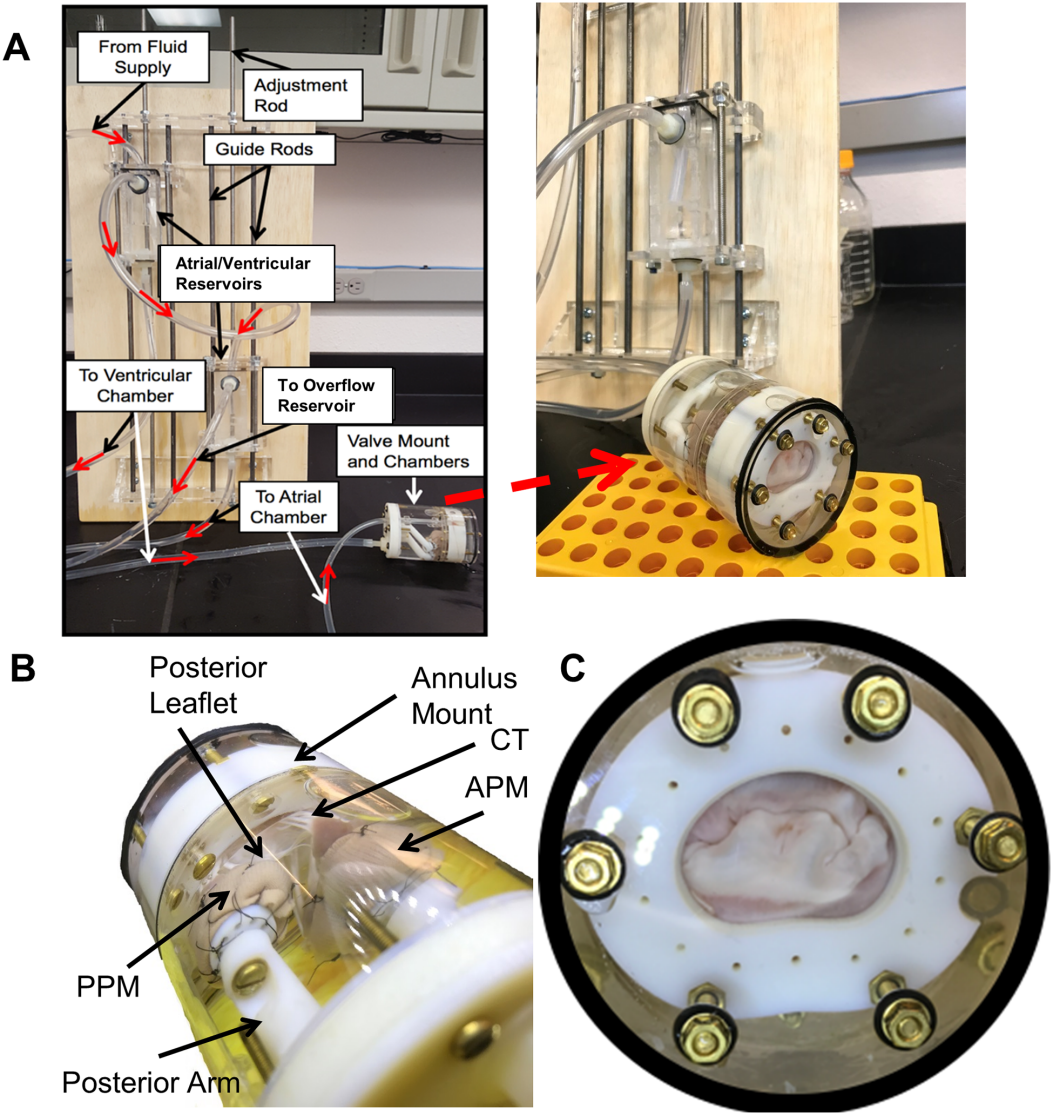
Pressure control system and valve pressurized in chamber. A) Control system as outlined in Fig 11, valve pressurized. B) Ventricular view of pressurized valve showing papillary muscle mounting. CT: Chordae Tendineae; APM: Anterior Papillary Muscle; PPM: Posterior Papillary Muscle C) Atrial view of pressurized valve showing a physiological leaflet coaptation pattern.
